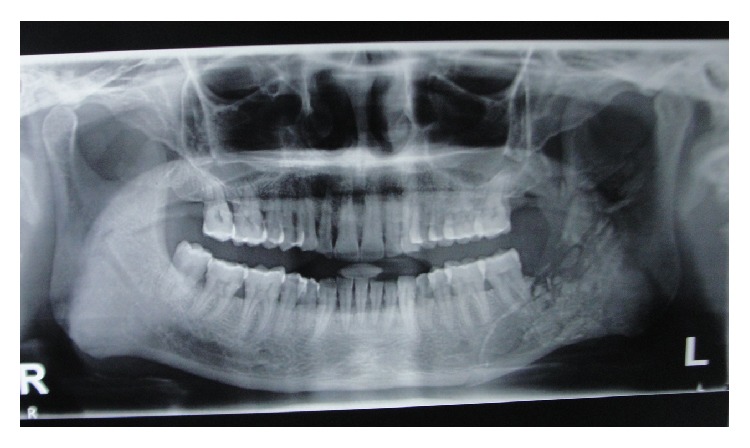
Orthopantomogram of the jaws three weeks after the injury.
